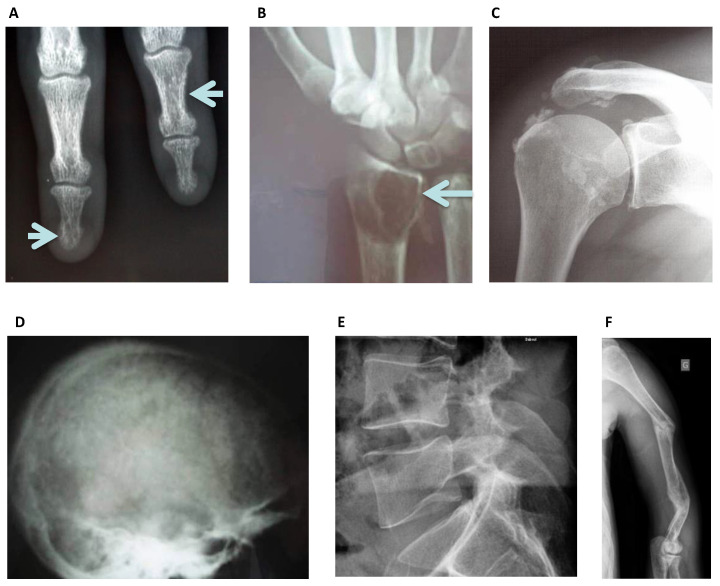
Standard Skeletal Radiography images: (A) Left lower arrow, sub periosteal resorption of the distal phalanx of the middle finger, and at the intermediate phalanx of the index finger as indicated by the right upper arrow in a hemodialysis patient, virtually pathognomonic of severe secondary hyperparathyroidism. (B) Image of a “brown tumor” at the distal radius metaphyseal as indicated by the arrow. It is a well-limited lytic lesion with endosteal scalloping, one of the possible manifestations of severe secondary hyperparathyroidism. (C) Periarticular calcifications of the glenohumeral ligaments, appearing as cloud-like densities that diffuse into the adjacent tenosynovial tissues. (D) “Salt and pepper” aspect of the calvaria seen as well-defined lucencies suggesting bone resorption. (E) Lateral spine X-ray can be used to assess vertebral fracture in a hemodialysis patient. (F) Multiple oblique spiral fractures in the proximal, middle and distal third of the humerus in a hemodialysis patient with osteomalacia.
